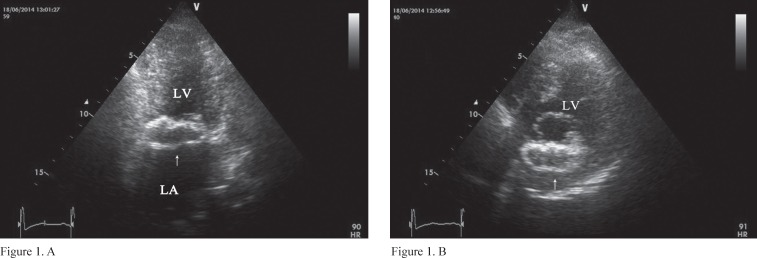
Echo-free space (arrow) in the posterior mitral annulus in the apical two-chamber view (A) and parasternal short-axis view (B).
